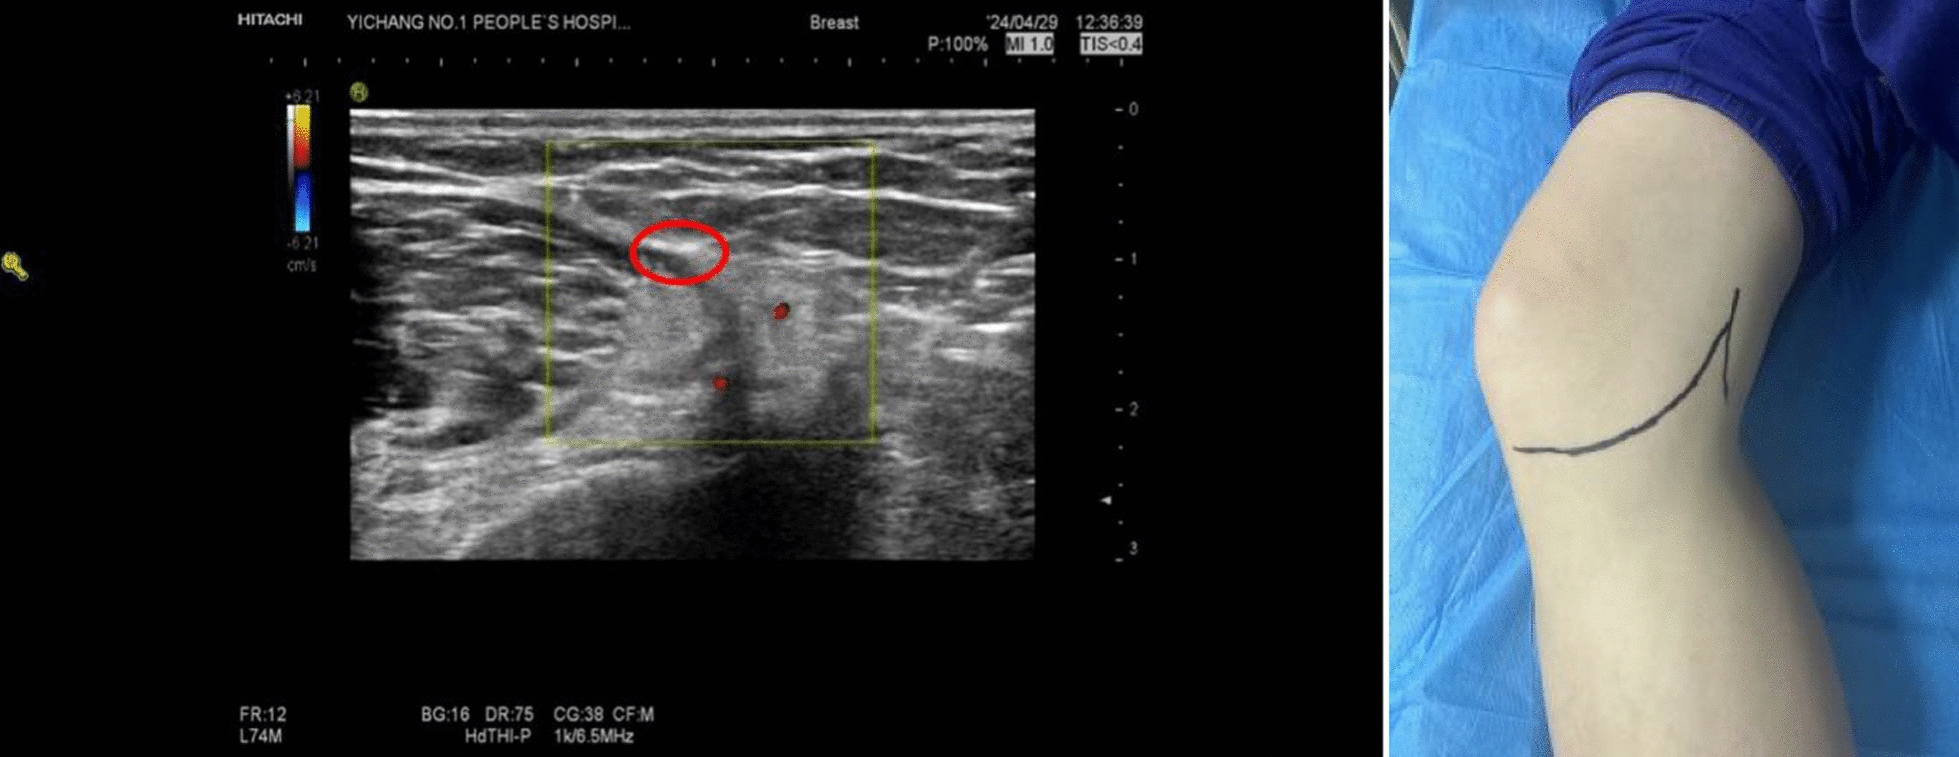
Preoperative ultrasound-guided localisation
